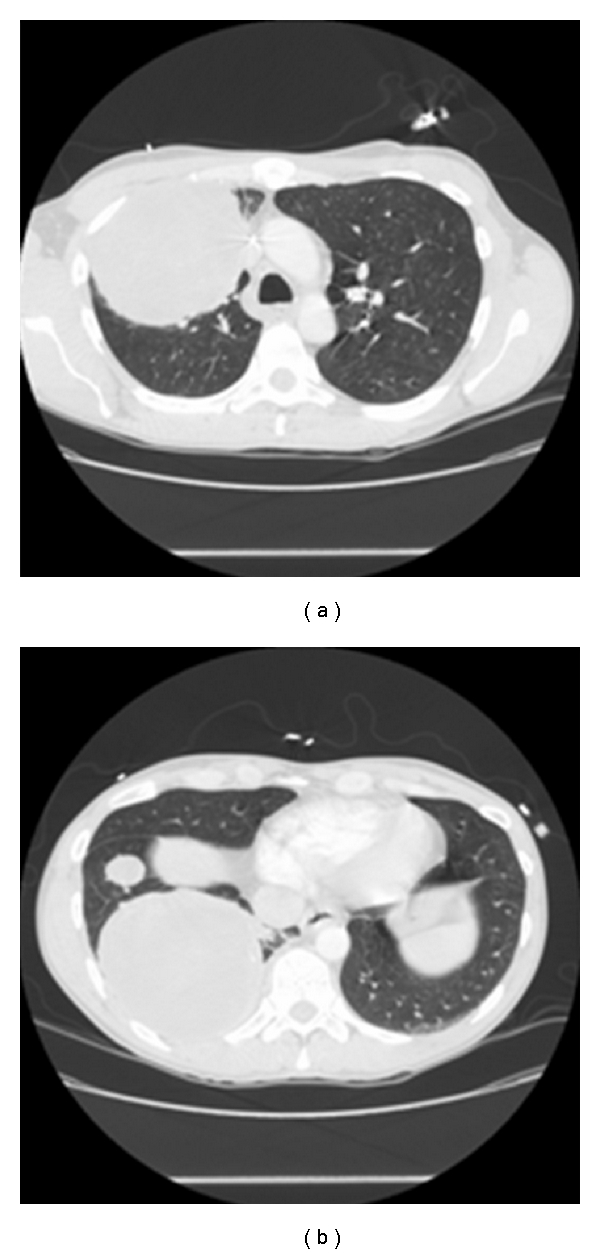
Chest computed tomography (CT) showed large right lung masses; the right upper lobe mass measured 6.8 cm, a large right lower lobe measured 10.5 cm.
